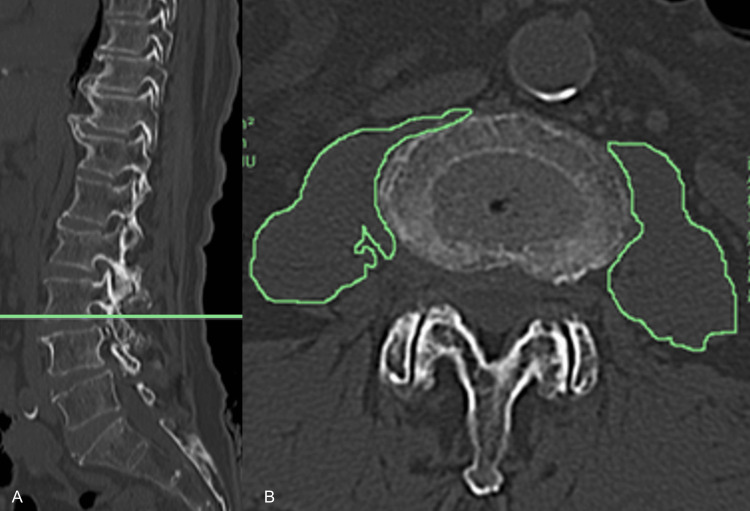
Measurement method of the cross-sectional area of the iliopsoas muscle Plain computed tomography of the thoracic and lumbar spine was performed upon admission. Panel A shows a sagittal view of the thoracolumbar spine, with a horizontal line drawn parallel to the inferior border of the third lumbar vertebral body. Panel B presents the corresponding axial image at the level indicated by the line in Panel A, in which the iliopsoas muscle was manually traced using the freehand method. All measurements were performed twice using the Picture Archiving and Communication System (Konica Minolta Medical & Graphic, Inc., Tokyo, Japan), and the average of the two values was used for analysis.
